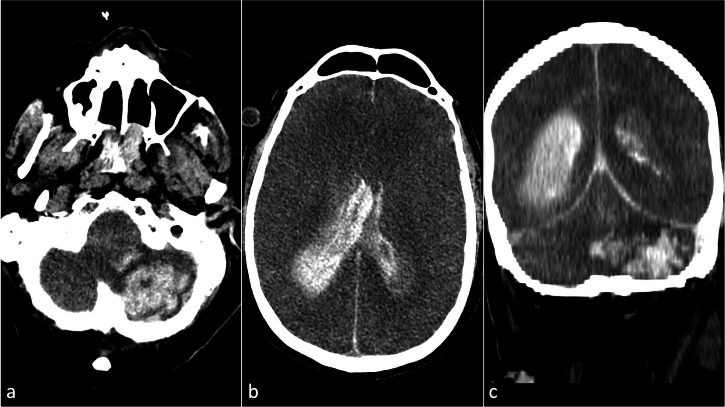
Unenhanced axial (a, b) and coronal images (c) computed tomography (CT) images in an extracorporeal membrane oxygenation (ECMO) supported patient show extensive spontaneous hemorrhage in the left cerebellar hemisphere (a, c), surrounded by vasogenic edema. Additionally, intraventricular hemorrhage is present in both lateral ventricles, along with a subdural hematoma along the falx cerebri and the tentorium cerebelli (b, c)
